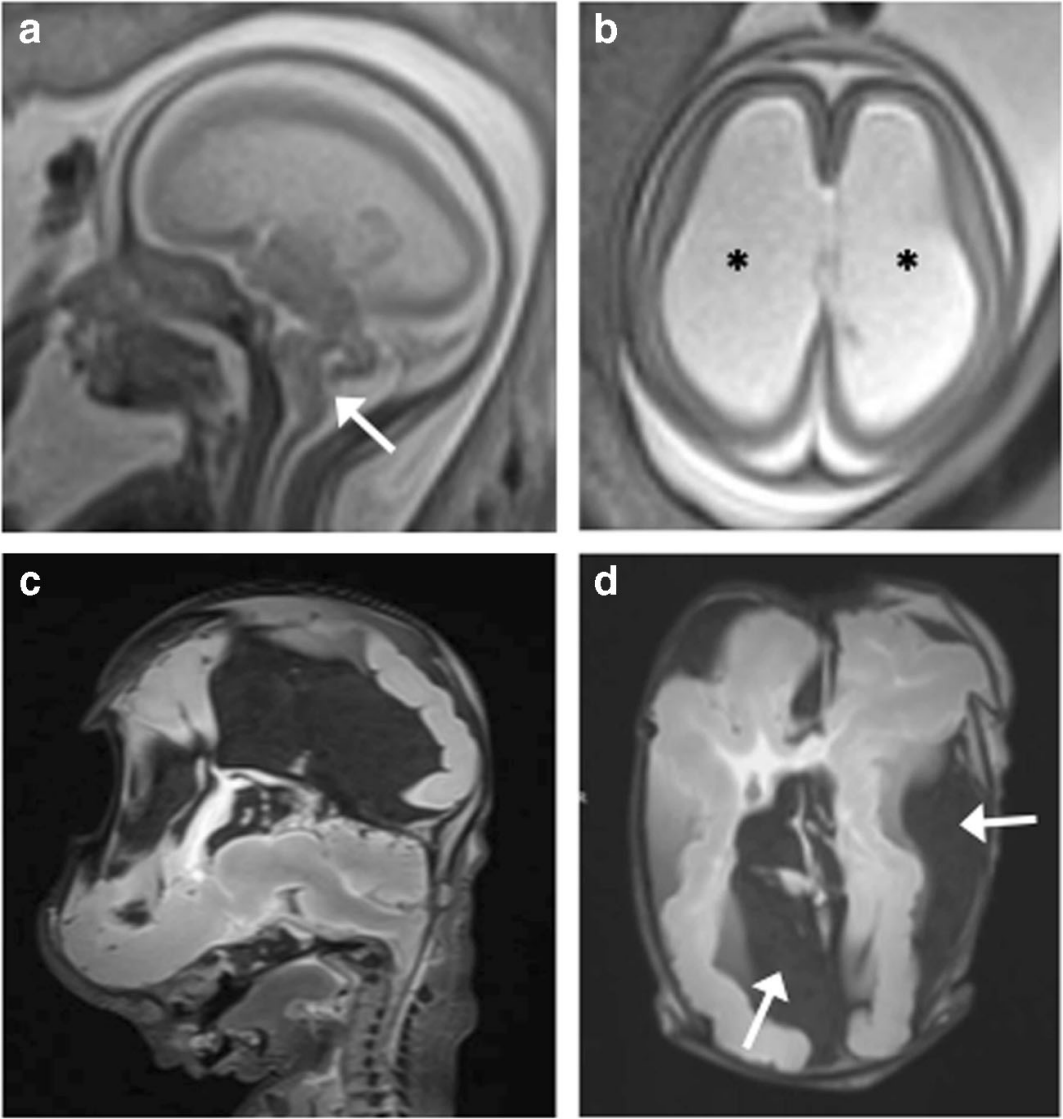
L1CAM (cell adhesion molecule) abnormality in a 30-week gestation fetus, with aqueductal stenosis. The fetus was also reported to have adducted thumbs on prenatal ultrasound examination. a Coronal and b axial T2-weighted iuMR images demonstrate kinking of the pontomesencephalic junction (white arrow), with severe ventriculomegaly (asterisks). The ganglionic eminences were not enlarged. c Sagittal and d axial T2-weighted PMMR following intrapartum cephalocentesis revealed collapsed ventricles and large extra-axial haematomas (white arrows). The haematomas were sustained during delivery but misinterpreted at PMMR as having been sustained prenatally
